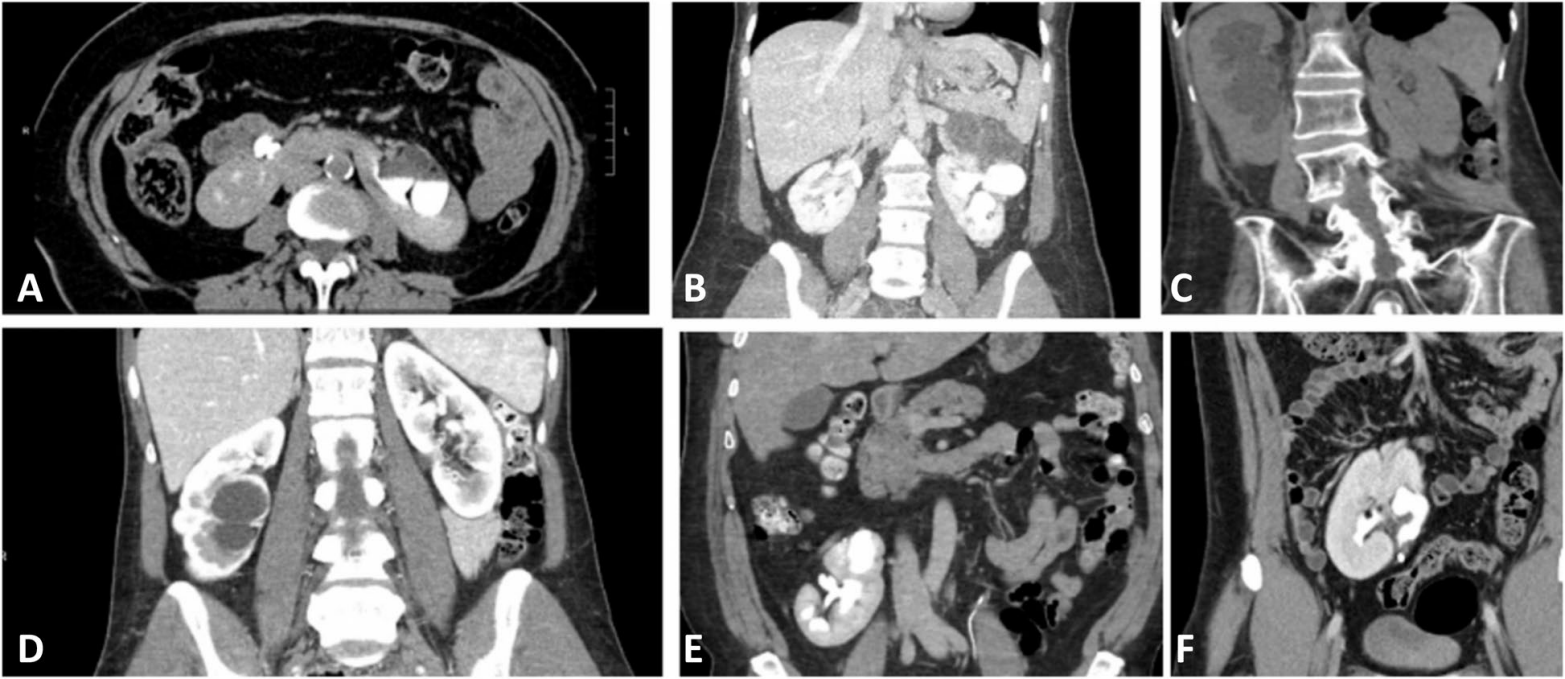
CT demonstrating various congenital renal anomalies: A Horseshoe kidney with left UPJO, B Duplex malrotated left kidney with upper pole hydronephrosis, C Right malrotated kidney, D Right sided duplicated system with lower pole hydronephrosis, E Ptotic malrotated left kidney, F Left pelvic kidney
